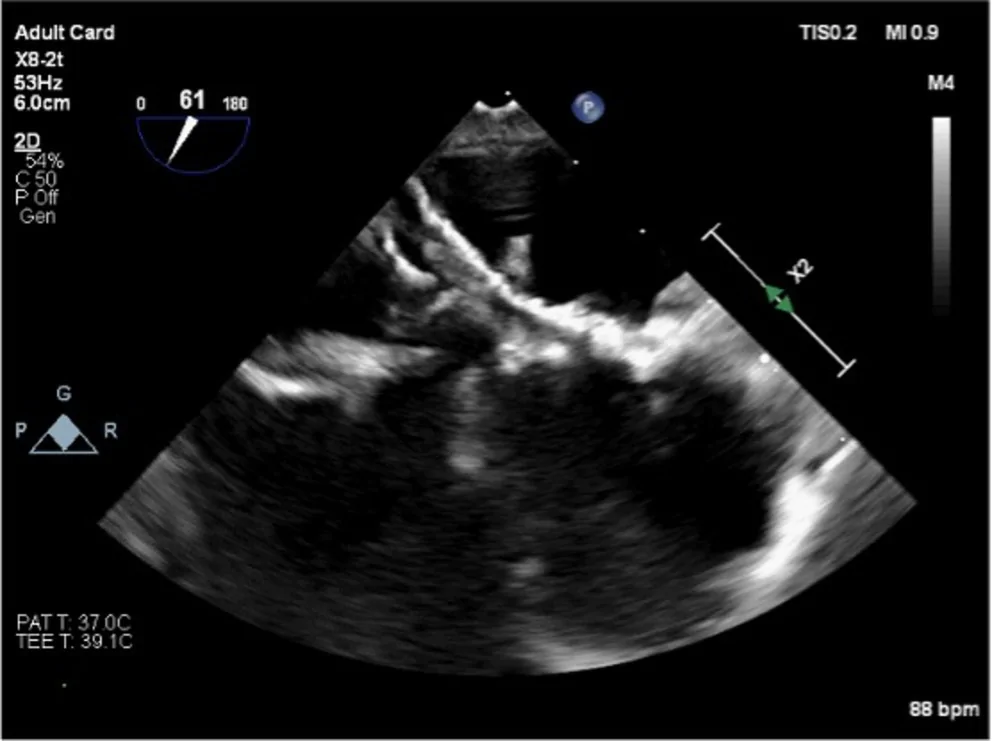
Transoesophageal echocardiography showing the device position after the release.
